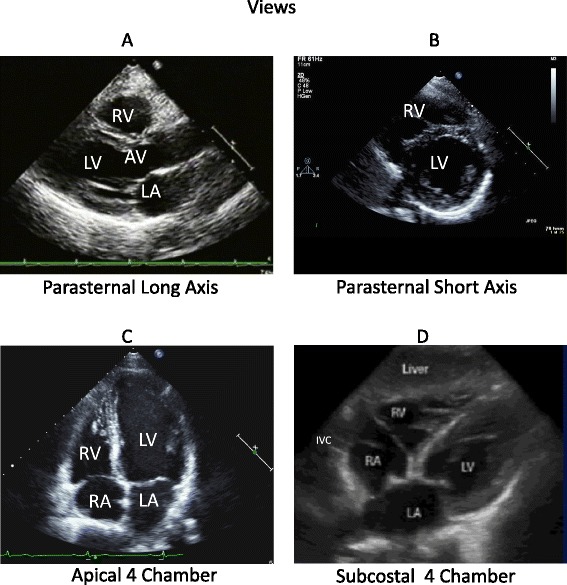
Standard four views of goal-directed echocardiography. (A) Parasternal long-axis view. Horizontal view of the heart, including the ascending aorta, the aortic valve (AV), the right ventricle (RV), the left ventricle (LV), the left atrium (LA), frequently excluding the apex, and the pericardium. (B) Parasternal short-axis view. A transverse view of the mid-LV at the level of the papillary muscles, including the RV, and the pericardium. (C) Apical four-chamber view, including the LV, RV at the RV inlet, trabeculated apical, and the infundibulum or smooth myocardial outflow regions, LA, right atrium (RA), mitral valve, tricuspid valve, and pericardium. (D) Subcostal four-chamber view. A more perpendicular orientation of the LV, RV, LA, RA, mitral and tricuspid valves, interatrial septum, pericardium, inferior vena cava (IVC), and liver.
